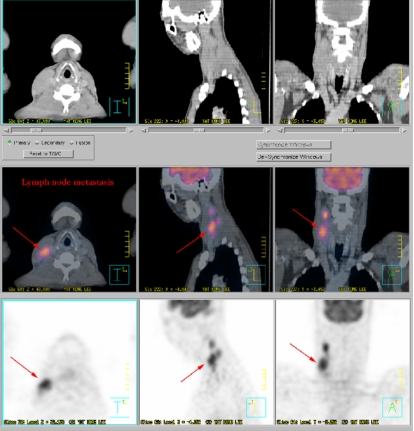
The fusion of CT and PET provide more accurate information for IMRT treatment planning. In this example, the spread of lymph mode metastasis of a nasopharyngeal carcinoma is can be clearly identified (courtesy of Dr. Hector Ma, St. Teresa's Hospital, Hong Kong)
